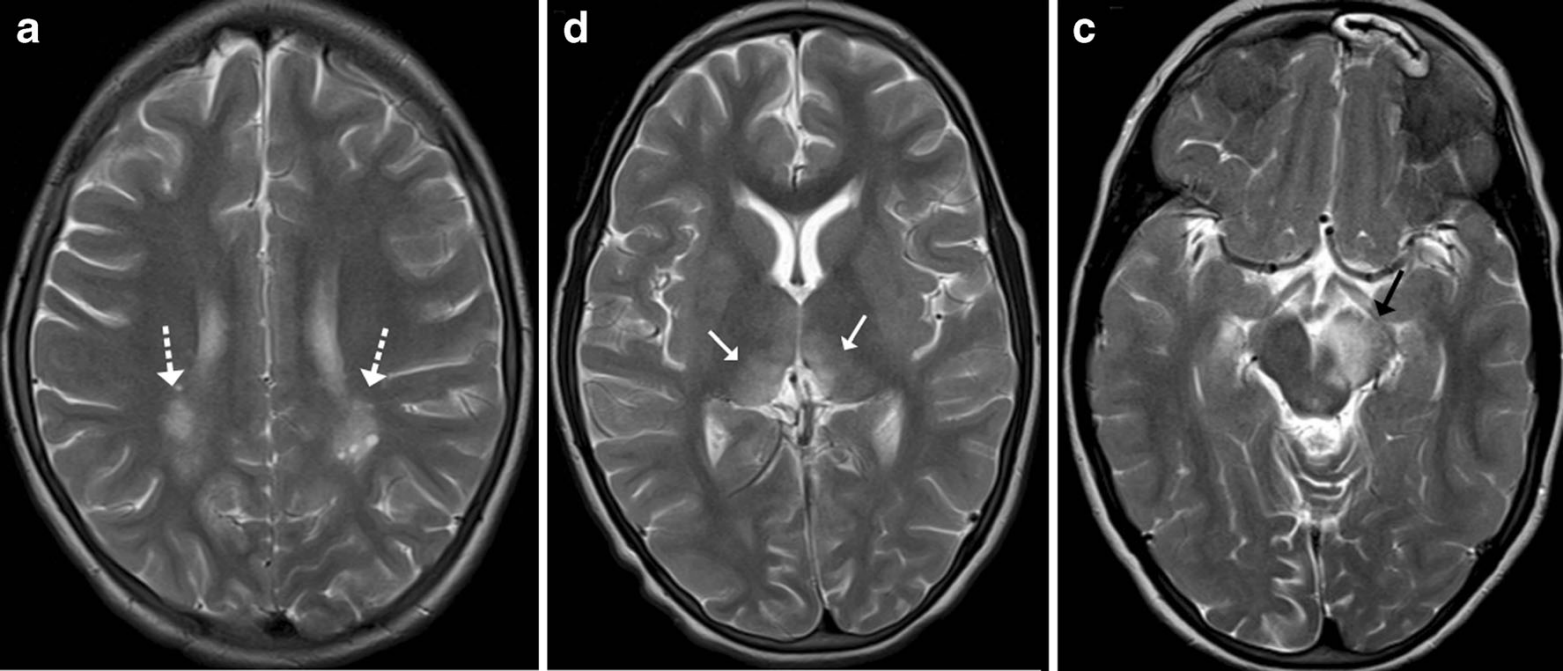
A 12-year-old boy with fever and seizures: a–c Axial T2-weighted images show hyperintense signal involving the periventricular white matter, medial thalamus and left middle cerebral peduncle suggestive of demyelination
